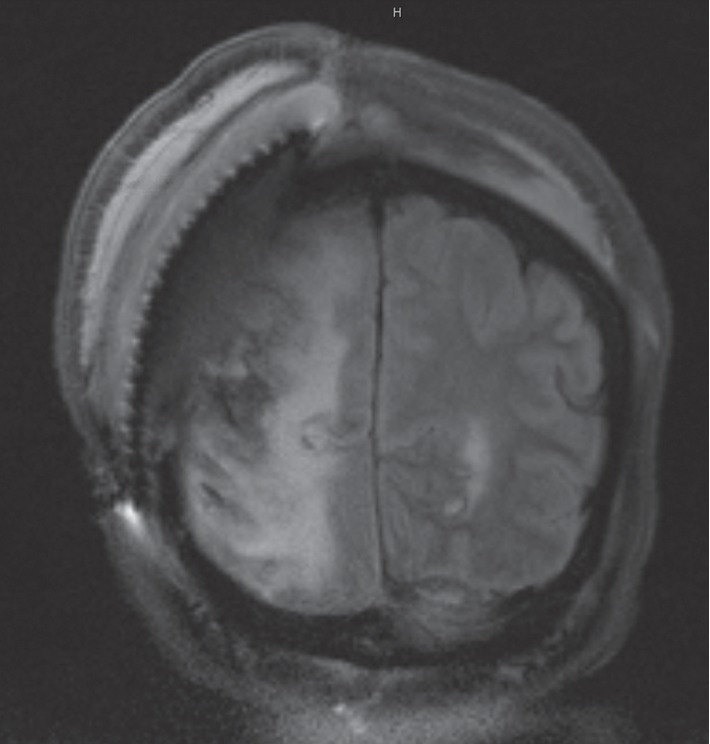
MRI of the brain demonstrating a decrease in the subgaleal fluid collection from 18 mm to 8 mm.
